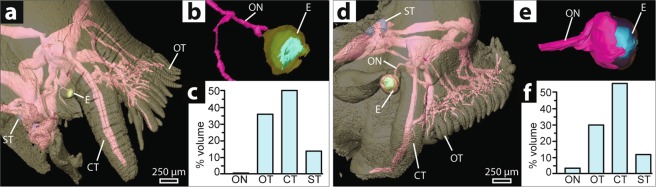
Sensory investment in *Elaphriella wareni* compared with *Ilanga navakaensis*. (a–c) *E. wareni* a reduced-eyed species. (d–f) *I. navakaensis* which has a typical vetigastropod eye. (a,d) Whole-head reconstructions. (b,e) Detailed reconstruction of eye. (c,f) Graph showing percentage of relative investment in sensory organs by volume for the optic nerve, oral tentacles and papillae (combined), cephalic tentacle and the volume of statocysts as a proxy for investment in this structure. Abbreviations: ON, optic nerve; OT, oral tentacles; CT, cephalic tentacle; ST, statocyst; E, eye.
